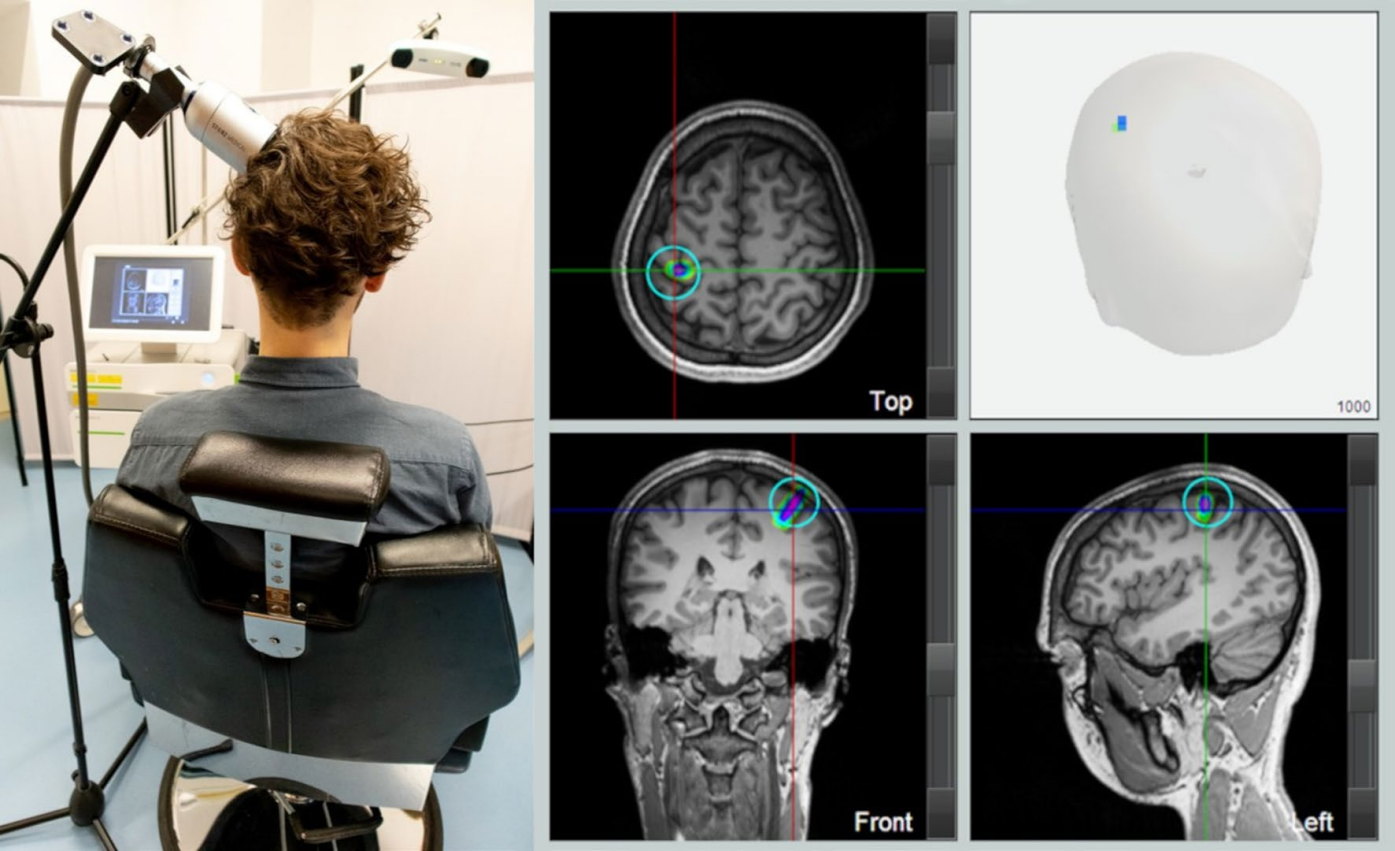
Focal transcranial pulse stimulation (TPS). TPS setup included a pulse generator device, a touch screen for real-time neuronavigation and an infrared camera system tracking the positions of the handpiece and the head of the participant via goggles affixed with infrared markers (left). The TPS handpiece was fixed using a tripod with a clamp focusing the ultrasound beam on the cortical primary somatosensory representation of the right hand, in the left postcentral gyrus posterior to the individual sigmoidal hook sign (marked by a turquoise circle). The TPS pulses of one session in a representative subject are displayed on the reconstructed head surface and in top, front and left orientation of the individual brain anatomy, showing the lowest (green) to highest pulse density (magenta)
